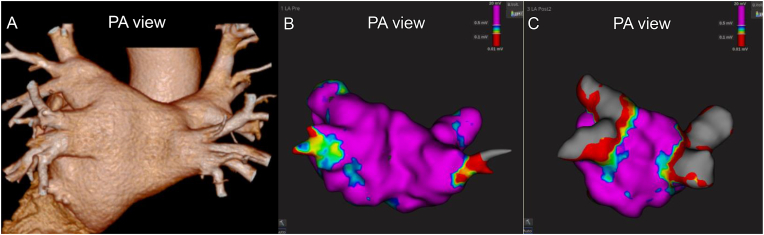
CT and three-dimensional mapping of the left atrium. (A) Pre-procedural three-dimensional CT of the pulmonary vein. (B) Pre-mapping using the Rhythmia system (Boston Scientific). (C) Post-mapping confirmed bilateral PVI after four PFA applications in the basket configuration and four in the flower configuration for each vein. PA, posterior–anterior; CT, computed tomography; PVI, pulmonary vein isolation; PFA, pulsed field ablation.
